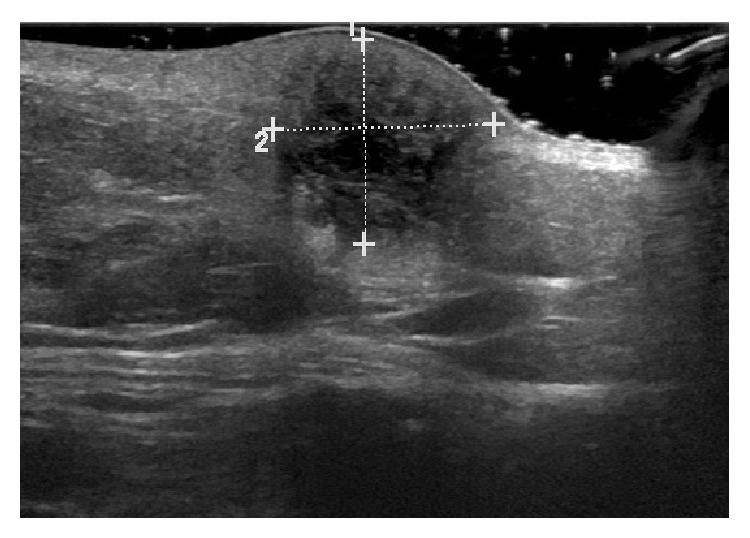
Ultrasonography image of the right breast showing 1.4 cm × 1.2 cm irregularly shaped, speculated, hypoechoic lesion with central necrotic changes and high vascularity.
